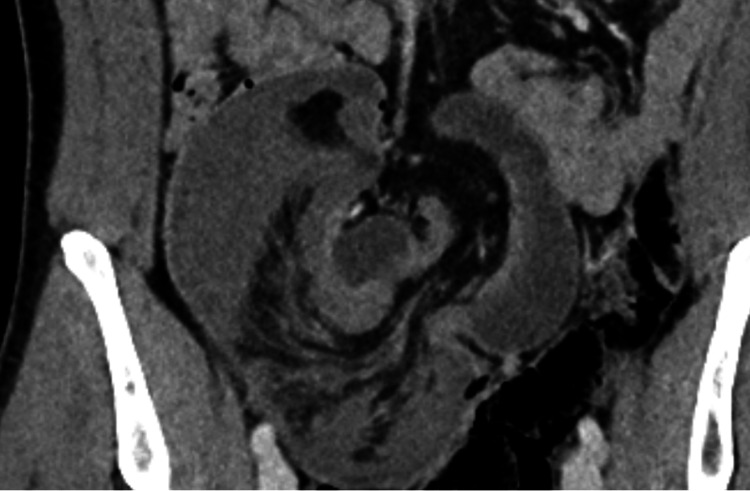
Ileocolic intussusception with hypoenhanced bowel. Coronal view of CT abdomen and pelvis, portal-venous phase.
